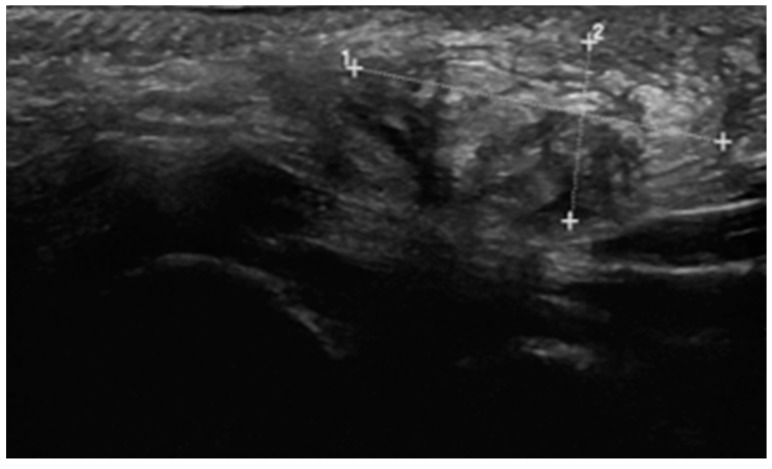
Sonographic findings evidenced a mass of 26 × 15 × 10 mm of diameters with heterogeneous echotexture.
